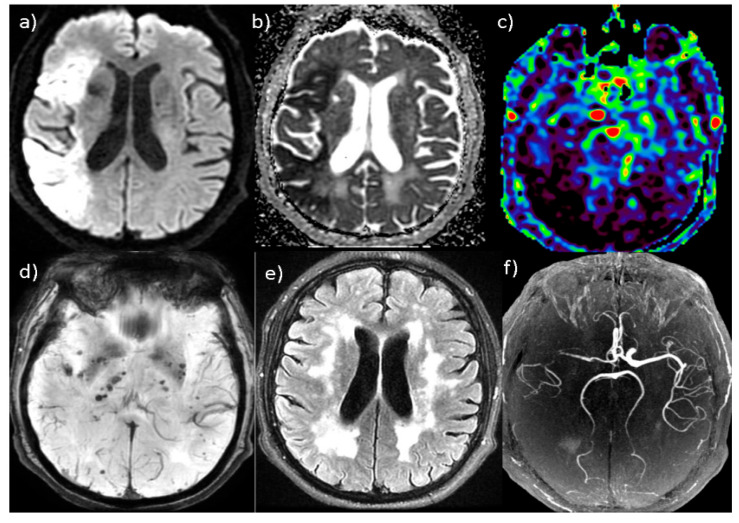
Brain MRI in hyperacute ischemic stroke patient: (a,b) axial DWI tomogram shows restricted diffusion in the irrigation of the right middle cerebral artery, with low ADC values; (c) 3D ASL perfusion revealed a hypoperfused right cerebral hemisphere; (d) axial SWAN tomogram detected multiple punctate cerebral microbleeds zones; (e) axial FLAIR tomogram shows large confluent white matter lesions; and (f) 3D TOF MRA shows occlusion of the right internal carotid and middle cerebral artery. MRI—magnetic resonance imaging; DWI—diffusion-weighted imaging; ADC—apparent diffusion coefficient; ASL—arterial spin labeling; SWAN—susceptibility-weighted angiography; FLAIR—fluid-attenuated inversion recovery; and TOF MRA—time-of-flight magnetic resonance angiography.
